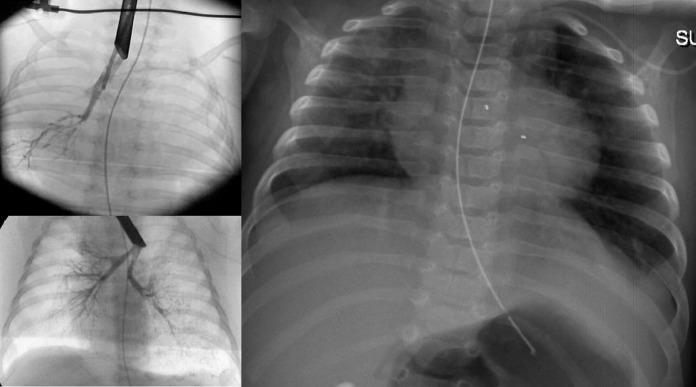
(Top left) Bronchogram prior to first stent insertion. (Bottom left) Bronchogram one day following initial stent insertion. (Right) Chest X-ray taken 10 days after stent insertion. Bronchial stent markers visible and nasogastric tube in situ.
